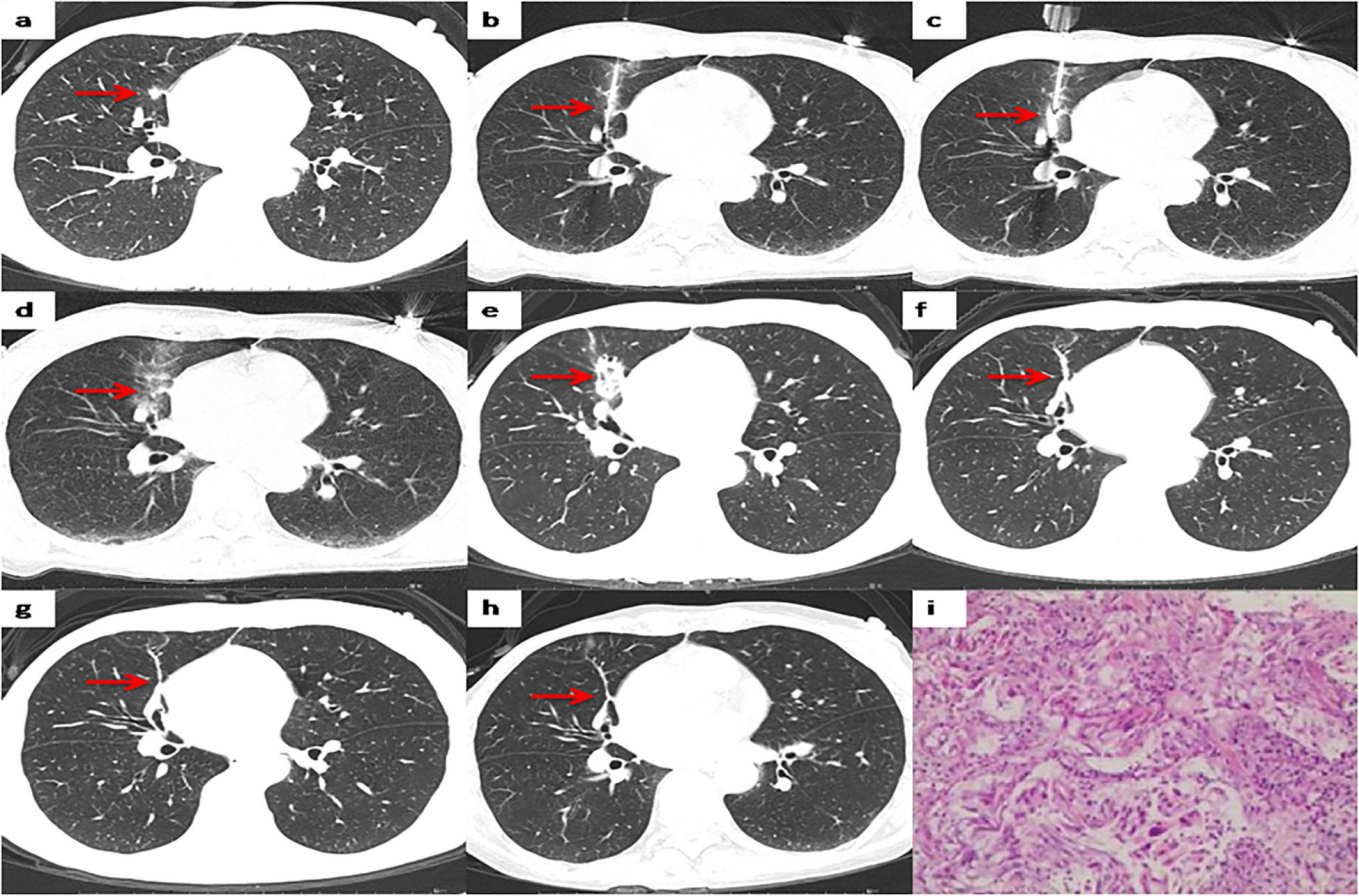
Representative CT scans of a patient with right lung metastases after breast cancer surgery. (a) Preoperative image. (b) The biopsy needle has been inserted into the nodule center to complete the biopsy sectioning. (c) The MWA antenna has been inserted into the nodule center. (d) Postoperative image immediately after MWA. (e) 1 month after MWA. (f) 12months after MWA. (g) 36 months after MWA. (h) 60 months after MWA. (i) Pathological results of lung metastases biopsy.
